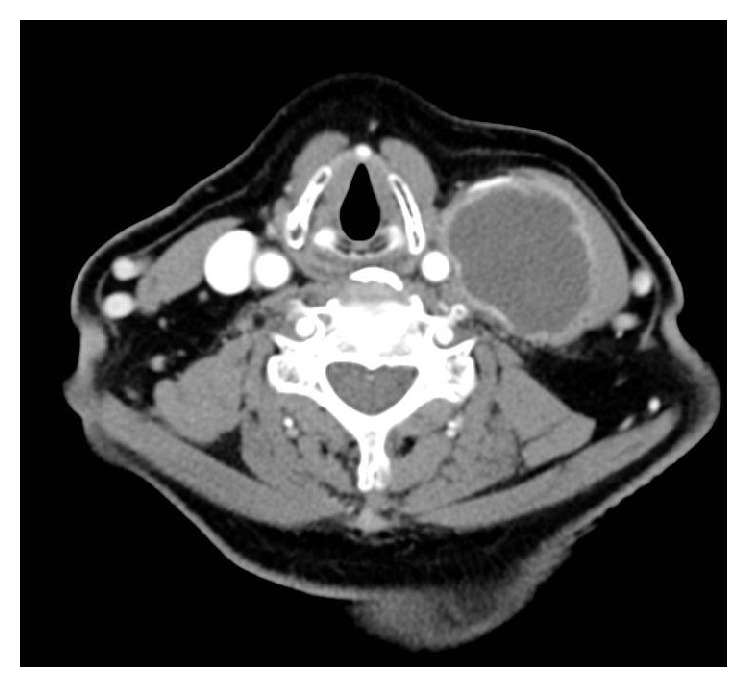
Computed tomography showing a 3.3 × 5.6 cm sized thick-walled cystic lesion.
